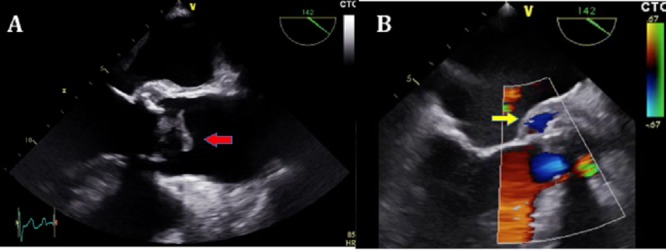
A. Transesophageal echocardiography demonstrating the large vegetation at the aortic bioprosthesis (15 x 23 mm; red arrow), B. Transesophageal echocardiography showing the aortic biological prosthesis and periannular aortic pseudoaneurysm (Doppler flow inside; yellow arrow).
